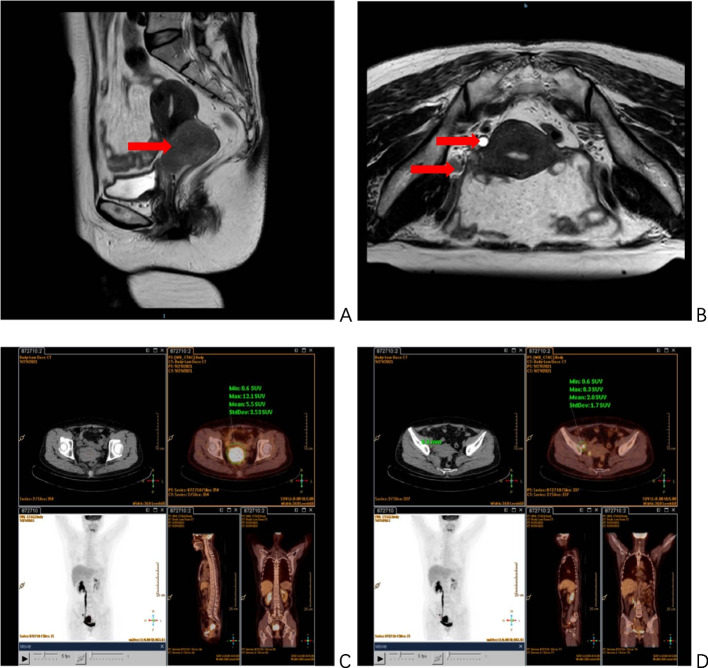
MRI before receiving treatment: A cervical occupation (arrowed), measuring about 4.5*4.6*4.8 cm, involving the external cervical os and poorly demarcated from the vagina; B an enlarged lymph node shadow is seen on the right side of the pelvic wall (arrowed); the right pelvic segment of the ureter is involved with fluid retention (arrowed). PET-CT before receiving treatment: C the cervix is significantly enlarged in size and metabolic activity, the lesion is poorly demarcated from the pelvic segment of the right ureter, with dilated ureter and renal pelvis hydronephrosis at its upper end; D metabolic activity of lymph nodes on the right side of the pelvic wall is increased, and metastasis is considered
